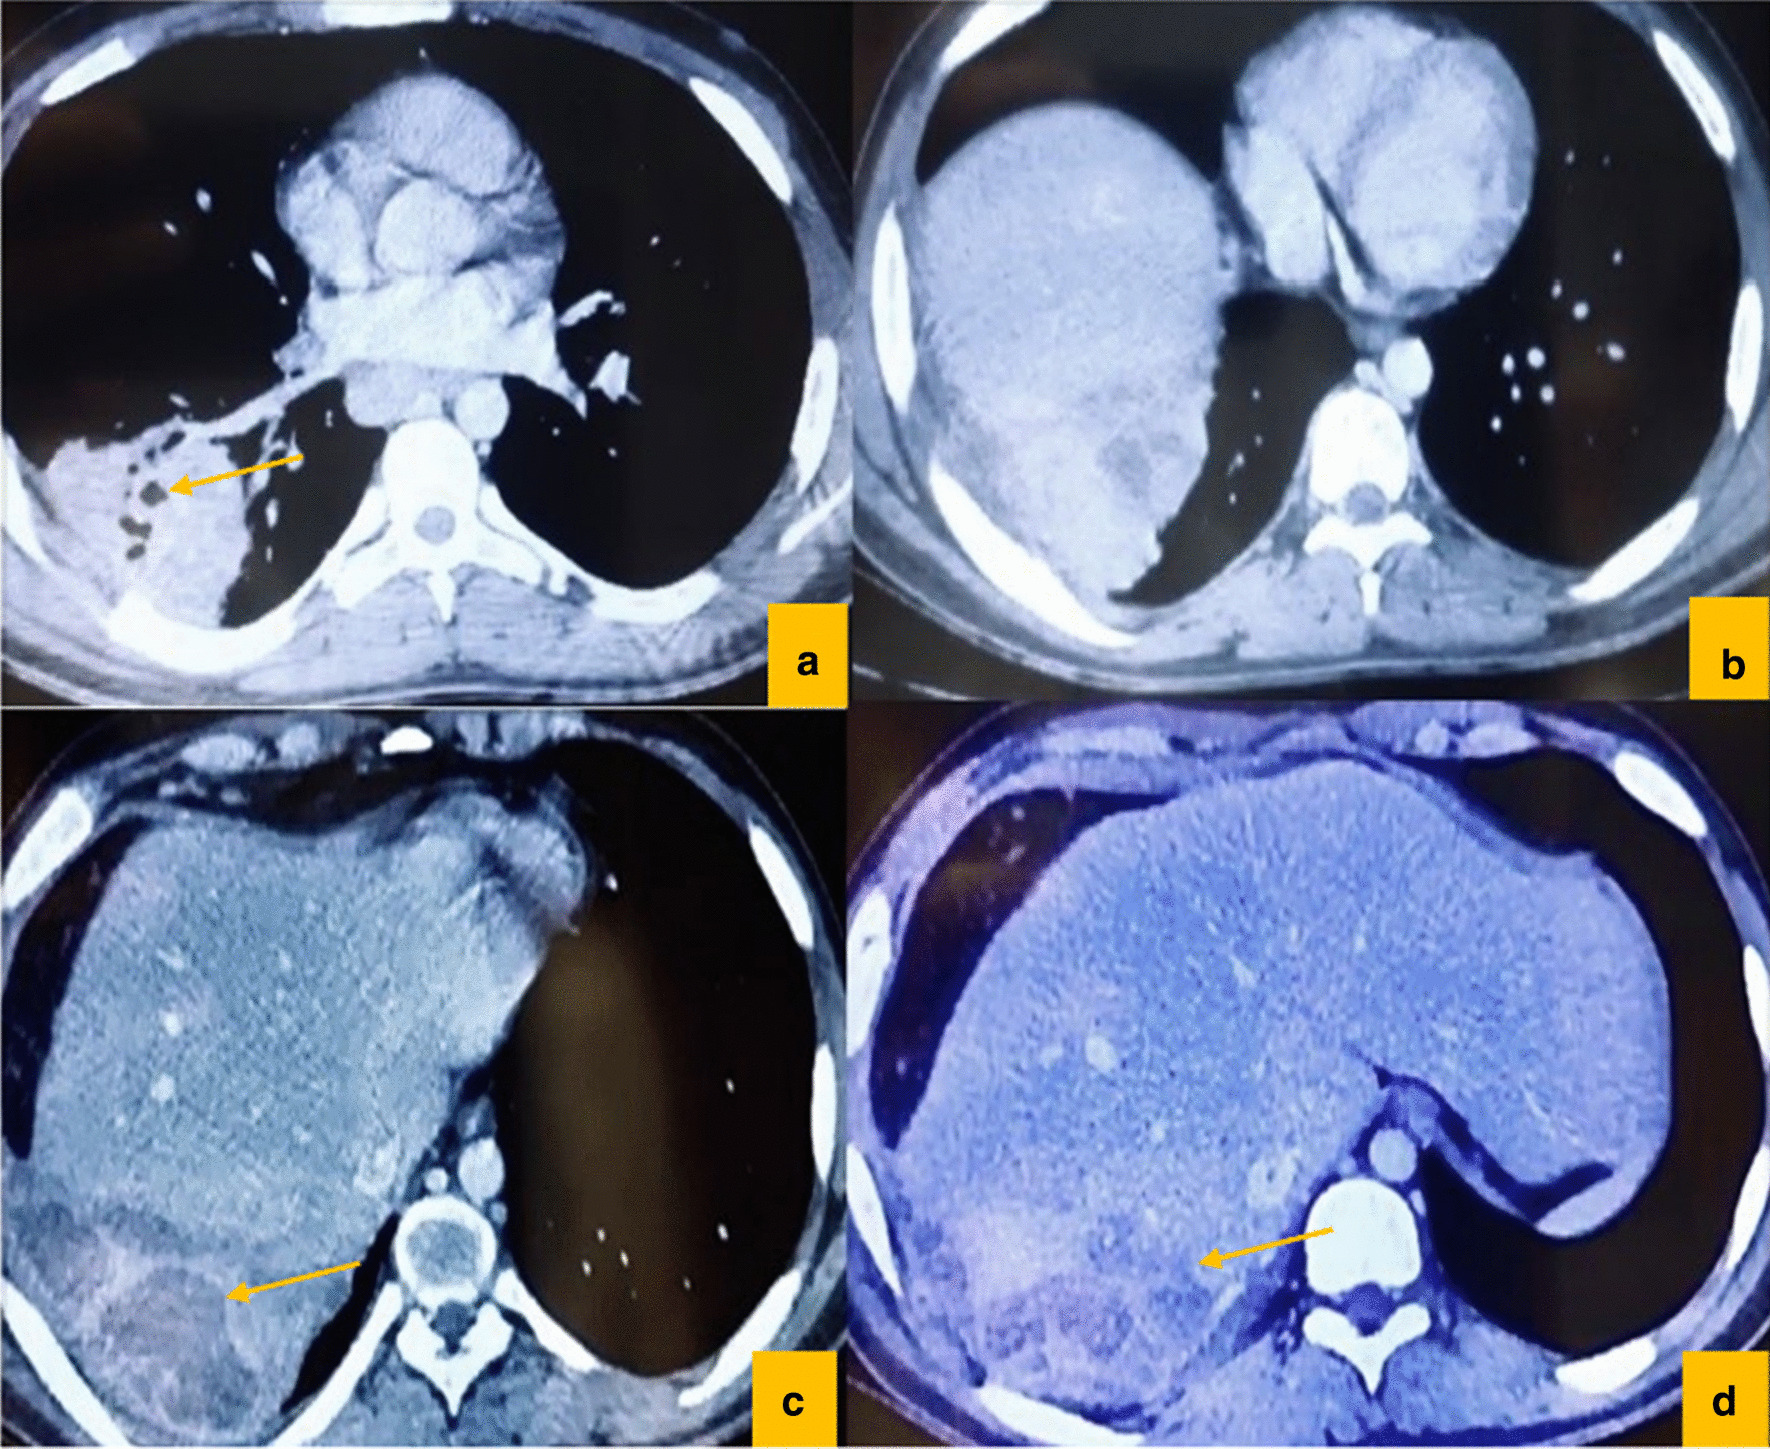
CT scan of thoracoabdominal region; arrow showing the right lung collection with air foci within the collection (a); arrow indicating the collection in segment VIII of liver (c) and (d)
